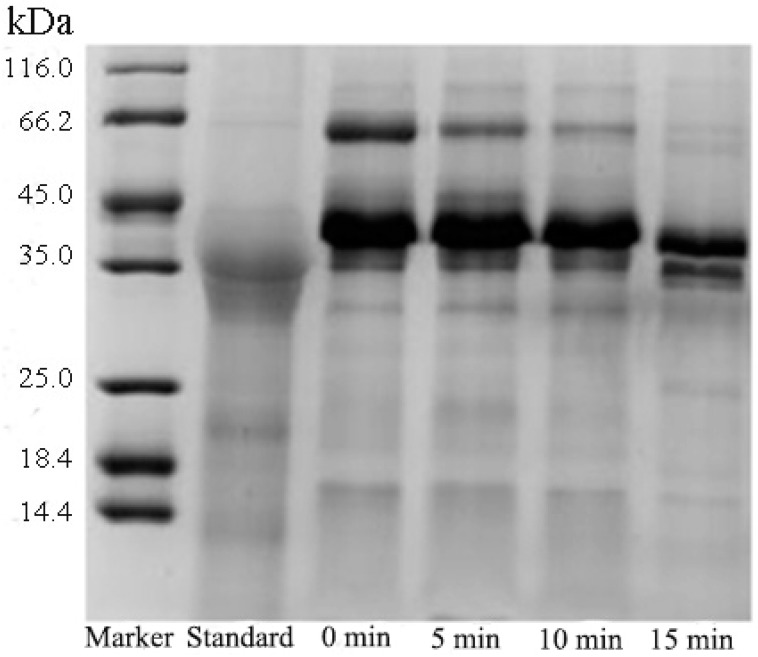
SDS-PAGE of Pv for different ultrasound times (0, 5, 10, and 15 min). Other operating conditions: Heating temperature, 80 °C; heating time, 15 min; and ultrasound power, 600 W.
